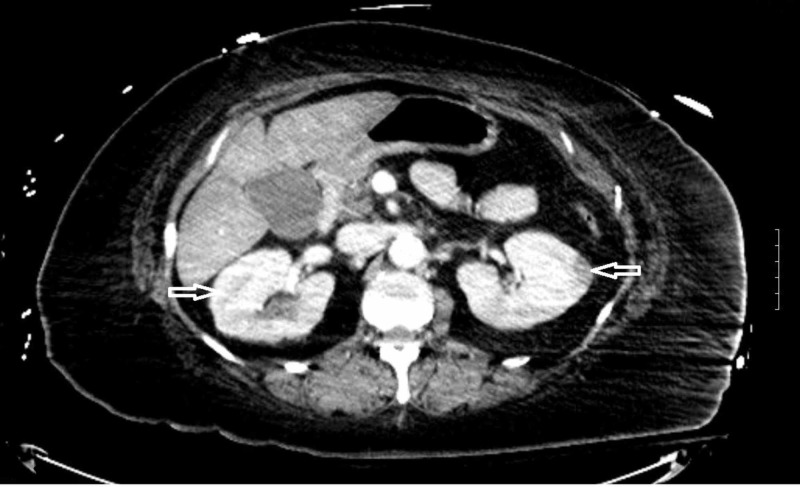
CT scan of the abdomen Cross-sectional CT image of the abdomen at the level of left renal artery with white arrows indicating patchy areas in both the kidneys.
